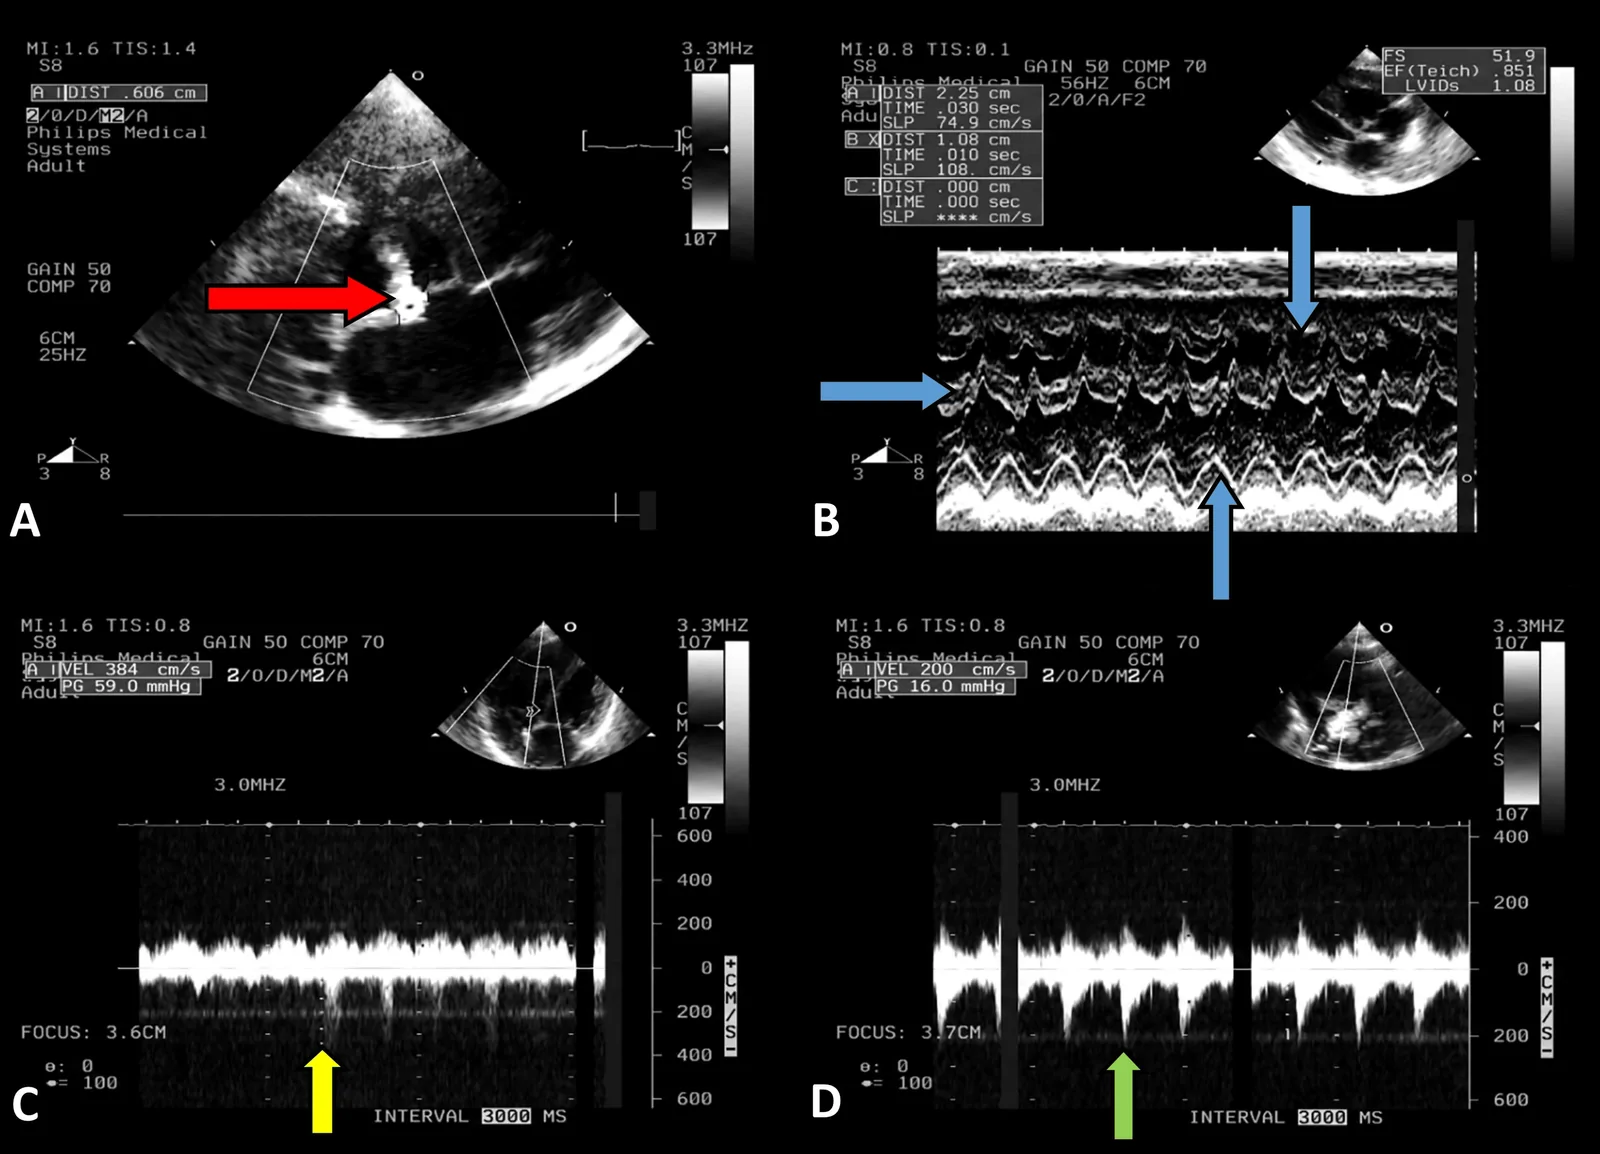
Preoperative echocardiographic assessment of left ventricular anatomy and hemodynamics. A: Two-dimensional parasternal short-axis view showing a large, non-restrictive PDA measuring 6.1 mm in diameter, indicated by the red arrow, permitting critical intercirculatory mixing. B: M-mode evaluation of the left ventricle demonstrating hyperdynamic baseline systolic function indicated by blue arrows marking measurement calipers. C: Continuous-wave Doppler interrogation of the tricuspid regurgitation jet revealing a peak velocity of 3.84 m/s (indicated by the yellow arrow), consistent with severe preoperative pulmonary hypertension. D: Continuous-wave Doppler of ductal flow showing a peak velocity of 2.0 m/s (indicated by the green arrow), confirming continuous pressure equalization.
